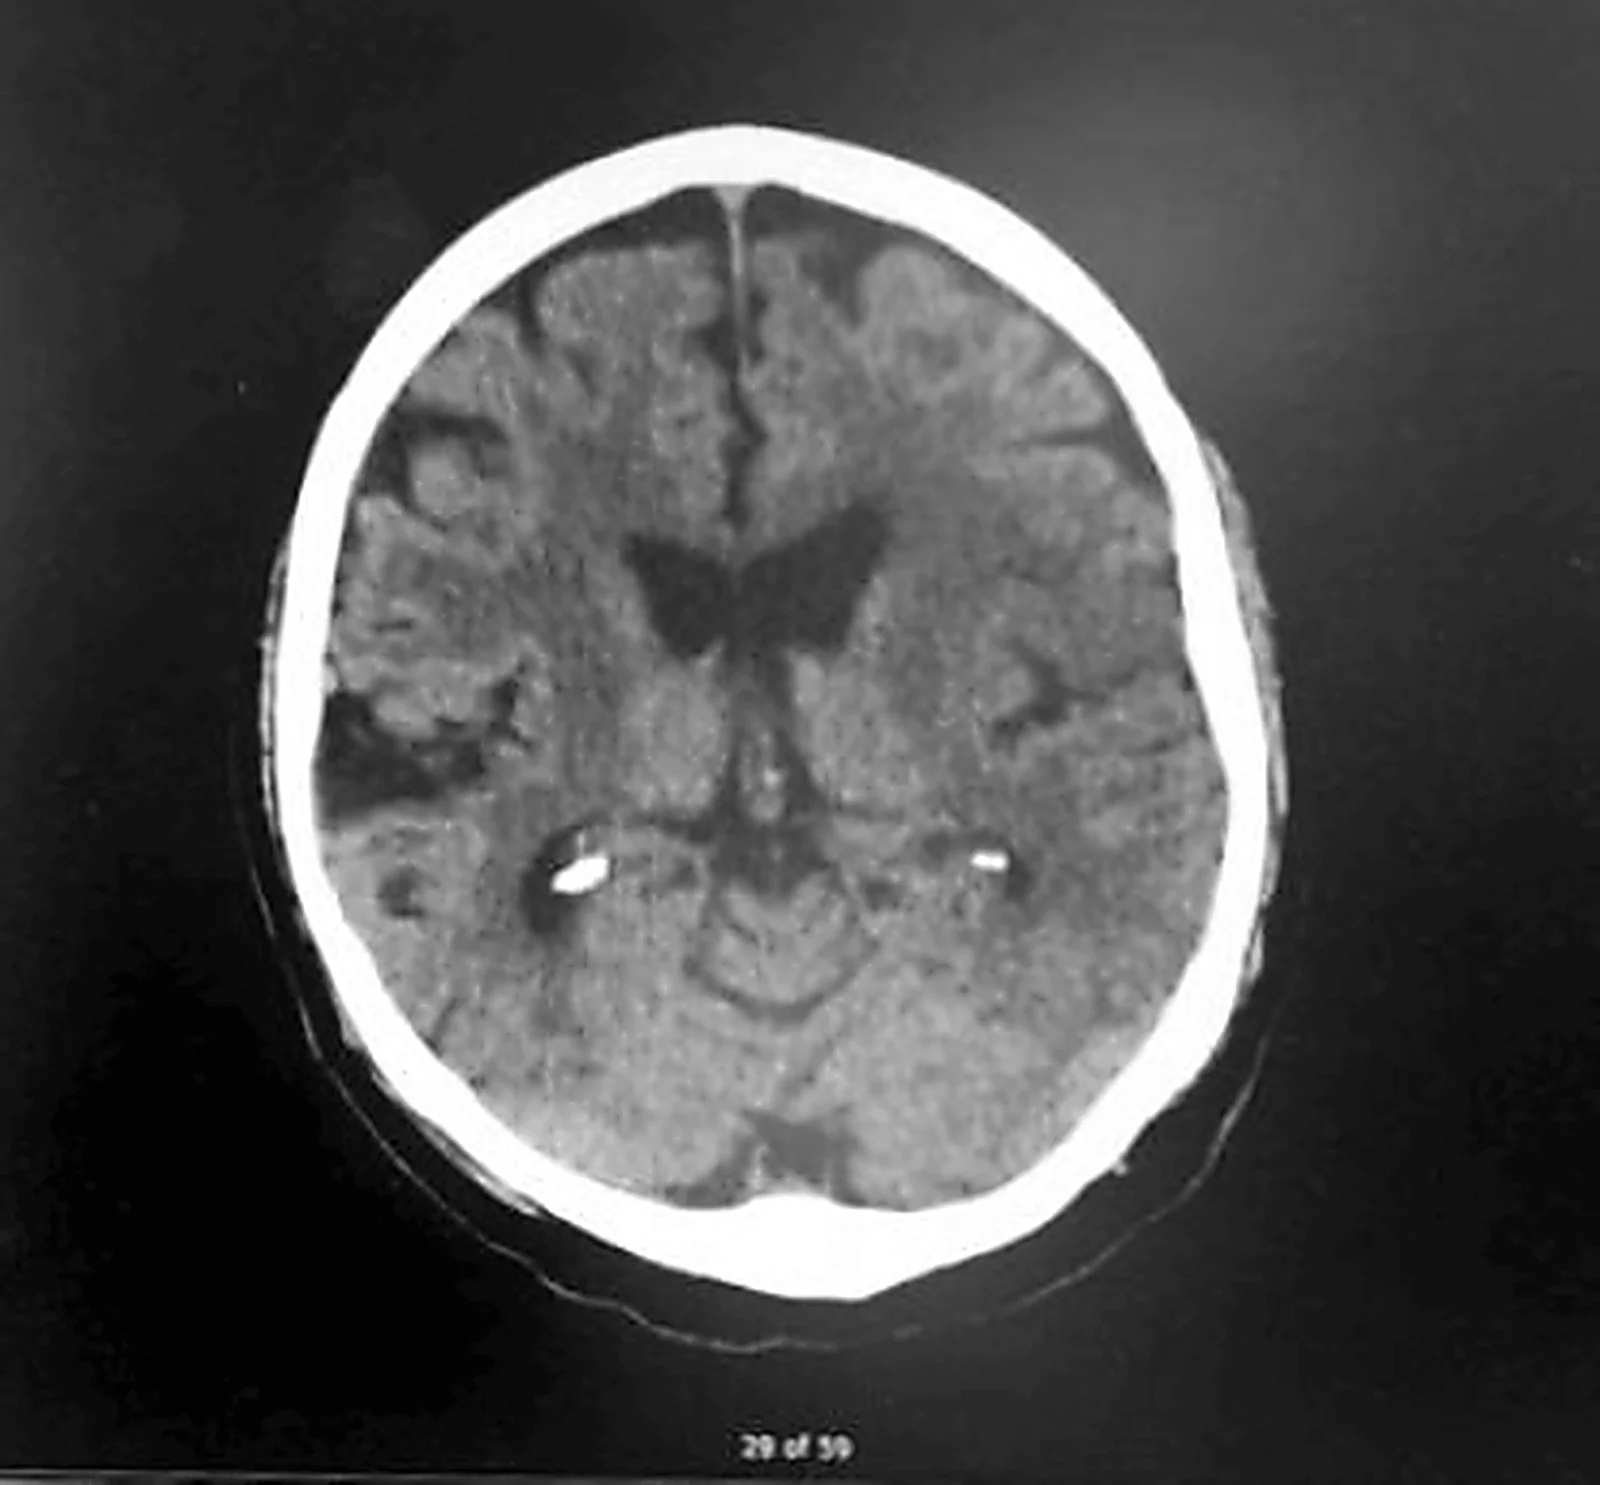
Non-contrast computed tomography (CT scan) No acute intracranial pathology identified; no evidence of intracranial hemorrhage, acute territorial infarction, space-occupying lesion, hydrocephalus, or midline shift.
